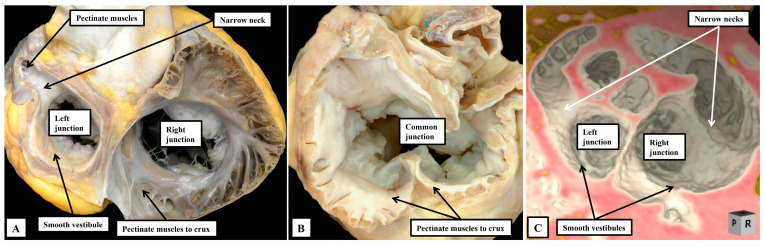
(A,B) have been prepared by cutting back the atrial myocardium so as to permit assessment of the atrioventricular junctions from above, with the left side seen to the right hand of the observer. (C) is a virtual dissection of a computed tomographic dataset producing a comparable view of the atrioventricular junctions. (A) shows the usual arrangement, with (B,C) showing the arrangements as seen when the atrial appendages are isomeric, with (B) showing right isomerism, and (C) left isomerism.
